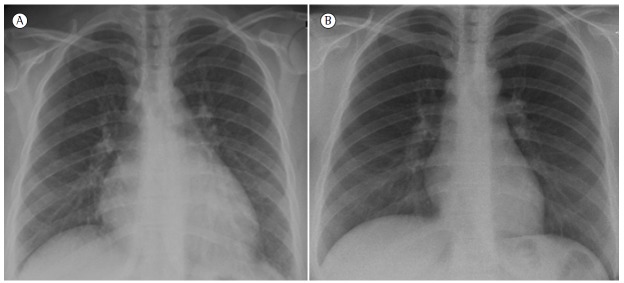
In A, a chest X-ray taken at admission showing a mixed interstitial
